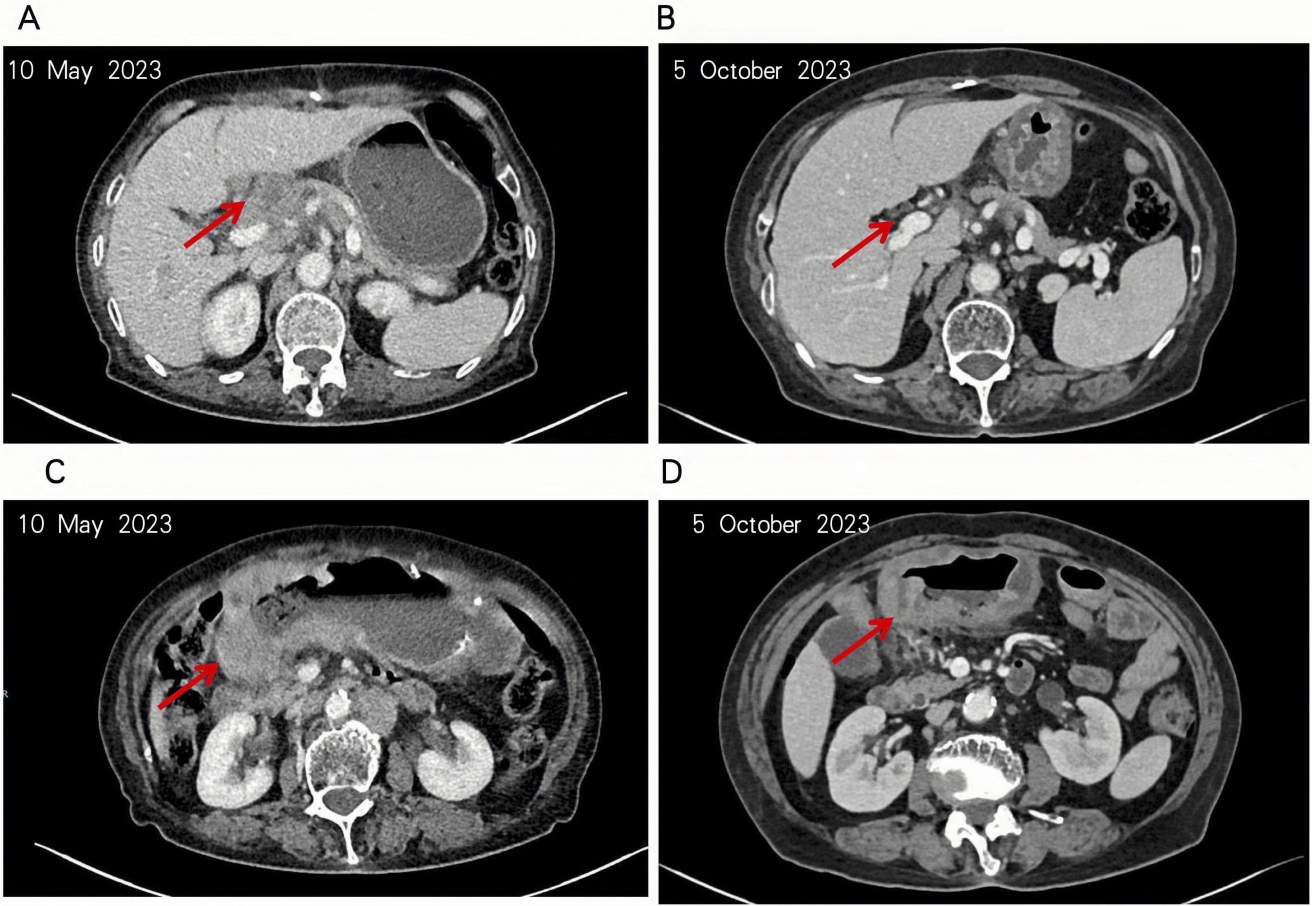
The patient’s tumor and hepatogastric interstitial lymph nodes were significantly reduced. (A, B) The patient’s lymph nodes in the hepatoportal and hepatogastric hiatus were significantly reduced as seen in the intravenous phase of enhanced CT. (C, D) Notable regression of the patient’s tumor in the gastric antrum observed in the same enhanced CT sequence.
